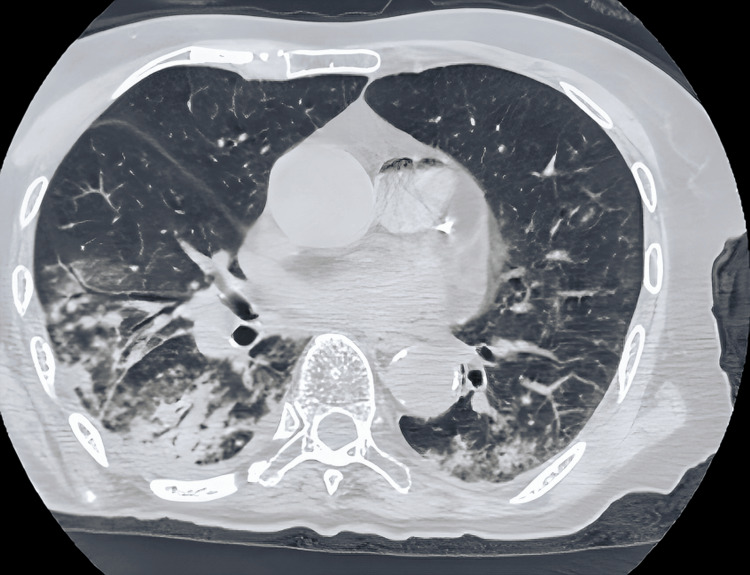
Chest CT at the onset of aspiration pneumonia (sixth day of hospitalization) Consolidation (arrow) and bronchial wall thickening are observed in the basal segments of both lungs. CT: computed tomography.
